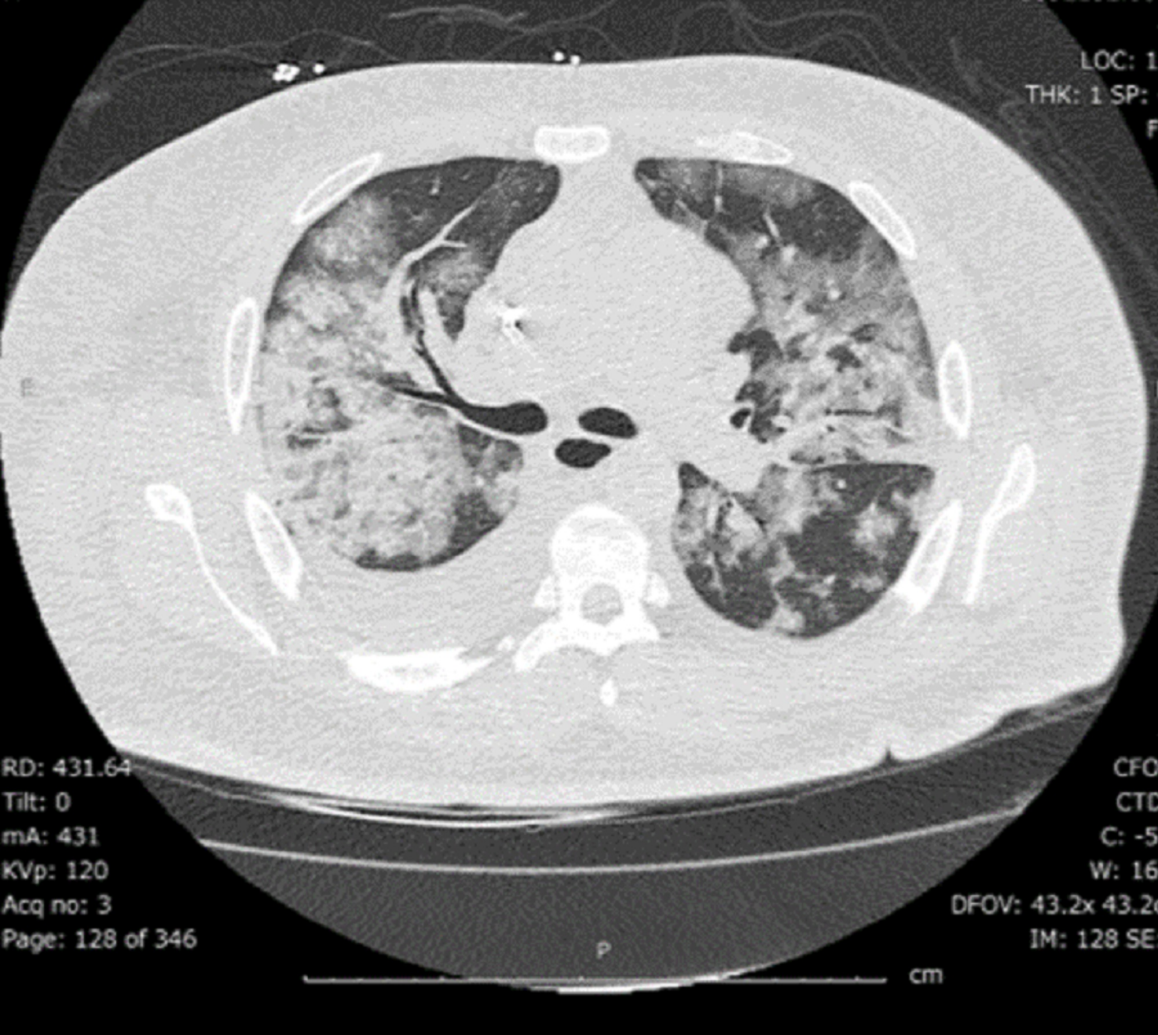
Computed tomography of the chest. Lung window showing typical B/L consolidative changes superimposed on ground glass changes (Day 3)
